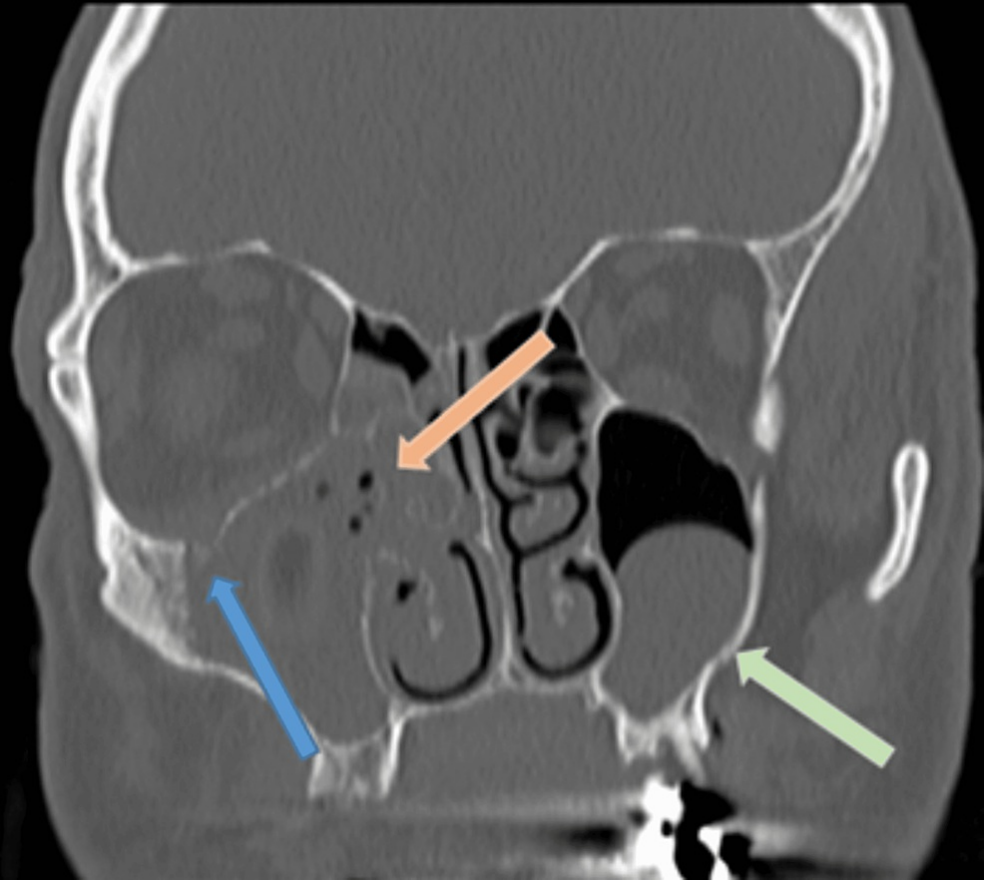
CT sinus – coronal image Adjacent subtotal osteomeatal unit opacification with anterior ethmoid and frontal sinus opacification (orange arrow) thinning and dehiscence of the medial sinus wall and orbital floor are visible. Again, a laterally positioned intra-sinus IOC is suggested (blue arrow). The well-defined polypoid mass in the left maxillary sinus is likely an incidental mucus retention cyst (green arrow).
